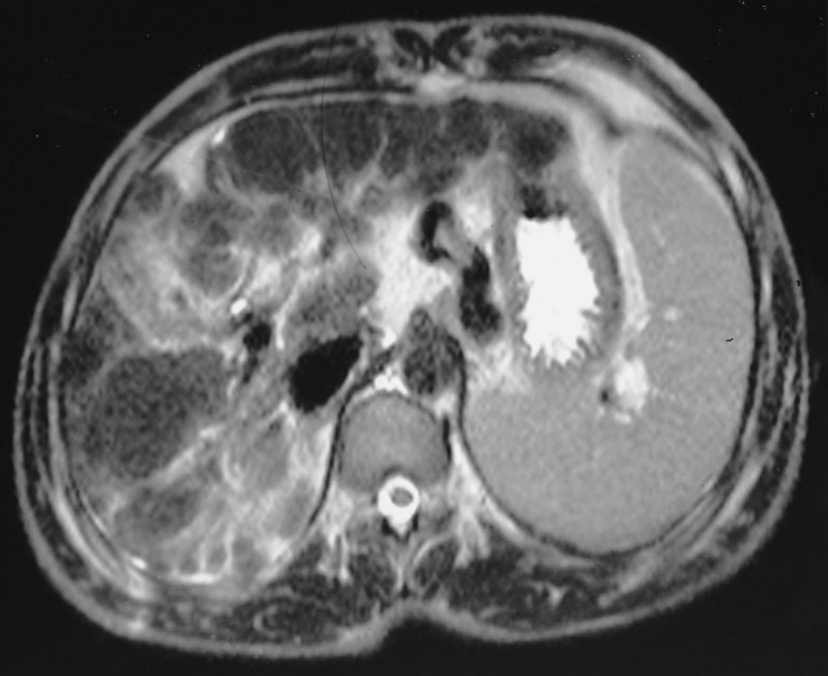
Magnetic resonance imaging showing multiple nodules in the liver
